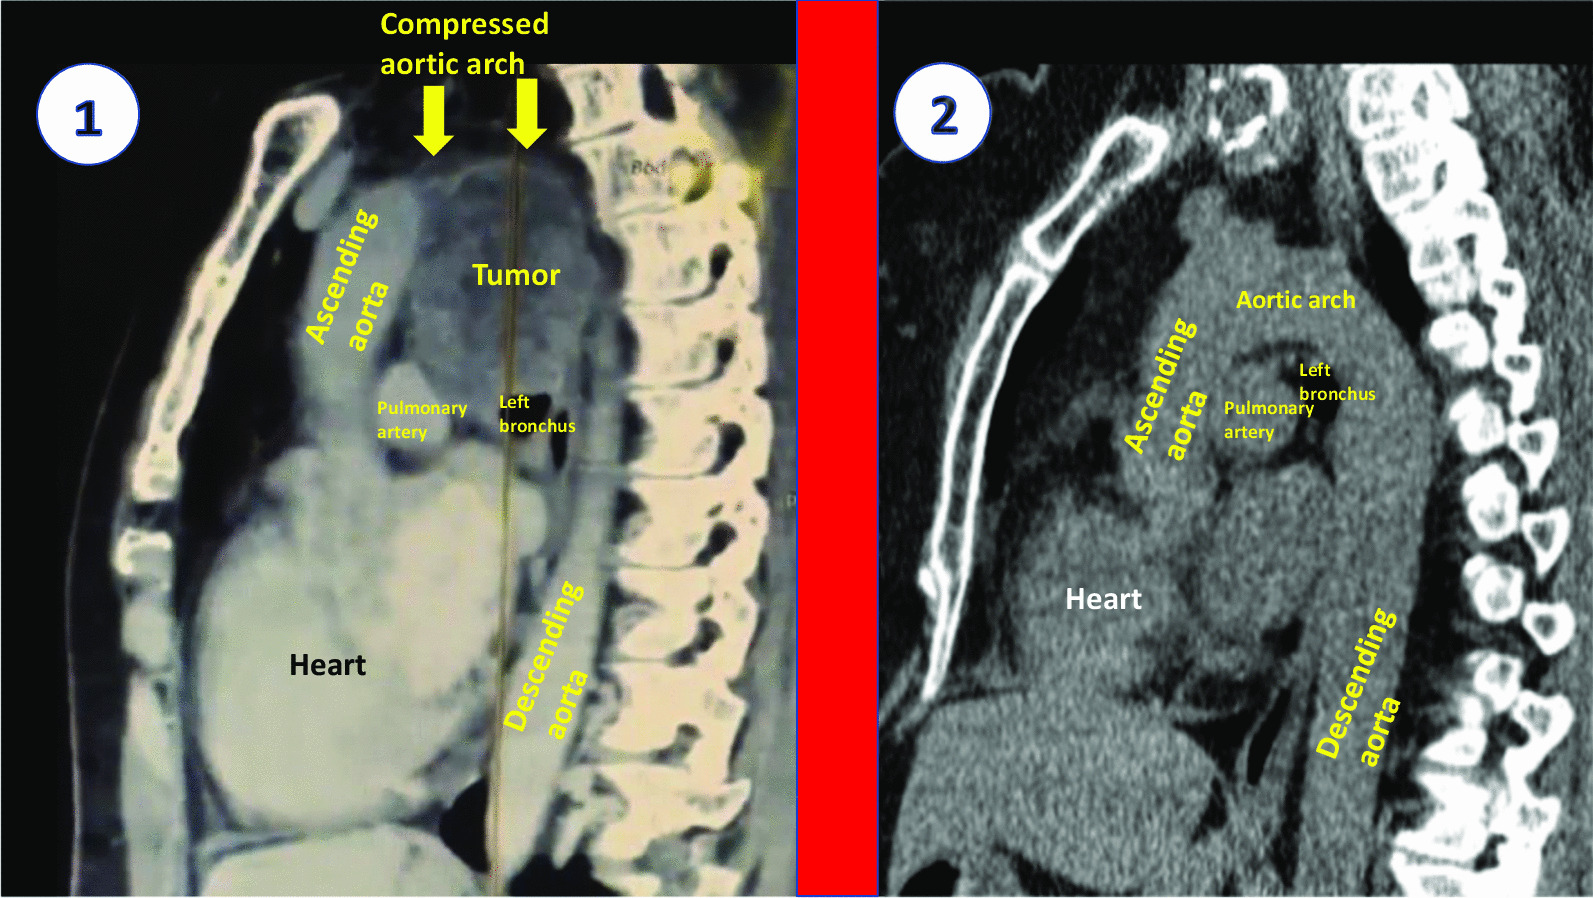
The chest computed tomography scan with a tumor of the mediastinum (1) and without pathology (2). The metastatic mass is circle-shaped, 77*43*65 mm, and heteroechoic. The tumor tightly abuts the aortic arch right by the course of the left recurrent laryngeal nerve.  Computed tomography scan of a different patient without any pathology of the mediastinum. The image is divided by a wide red line into two parts. All the important structures are marked with yellow, white and black inscriptions
